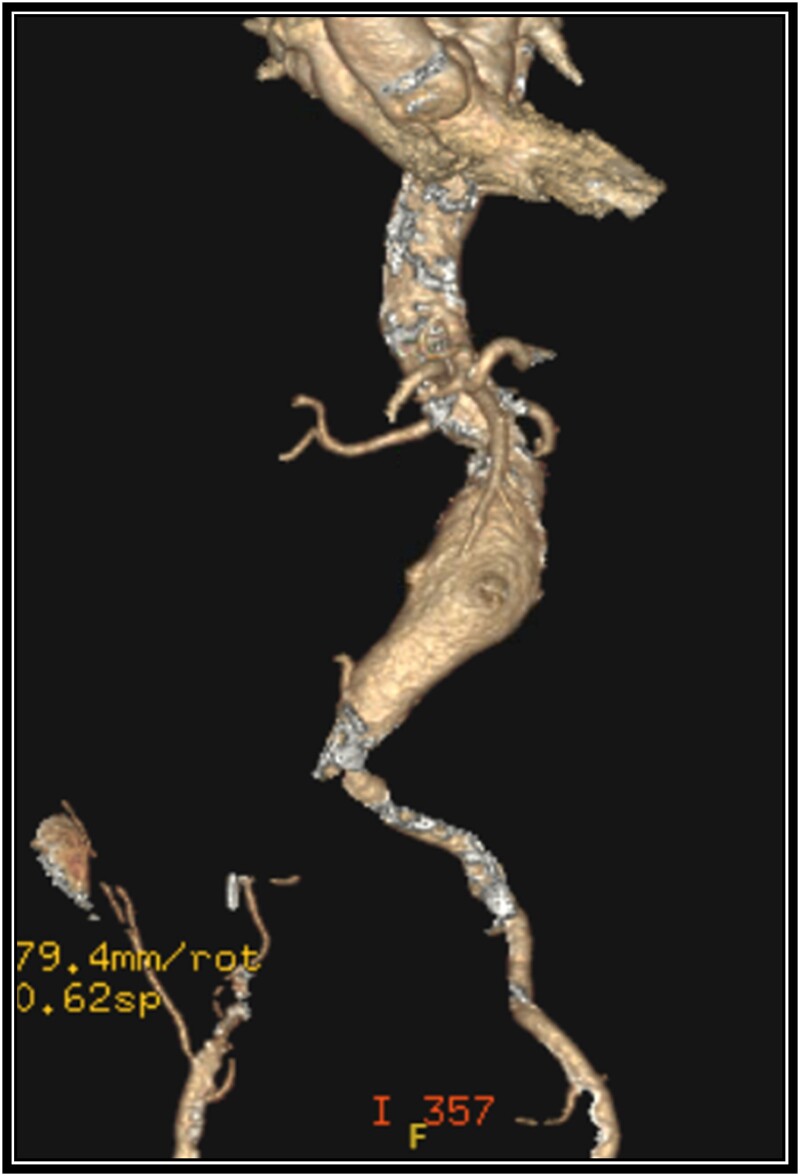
Preoperative 3-D reconstruction based on CTA imaging demonstrating an infrarenal abdominal aortic aneurysm and complete occlusion of the right common iliac artery with distal reconstitution.
